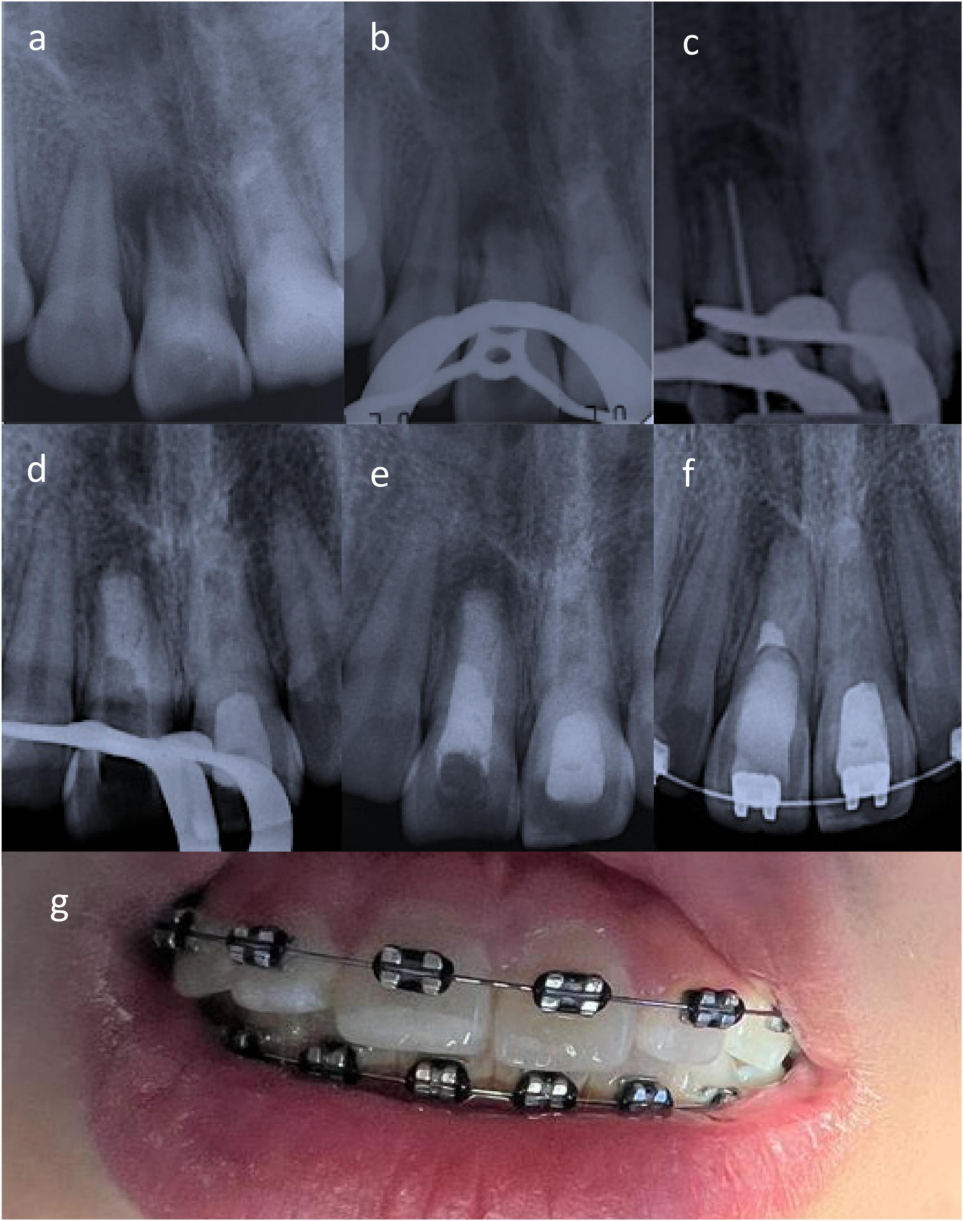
(a) Apexification induction of tooth 1.1 was indicated given the low likelihood of apical papilla cell presence due to the surgeon's curettage. (b,c) Upon removal intracanal medication suppuration was noted. (d,e) An apical plug with bioceramic cement and thermoplastic gutta-percha (apexification) was performed. (f) Radiographically, periapical repair was evident in teeth 1.1 and 2.1. (g) Clinically, the patient was in optimal conditions, with no clinical symptoms and restored teeth undergoing orthodontic treatment.
